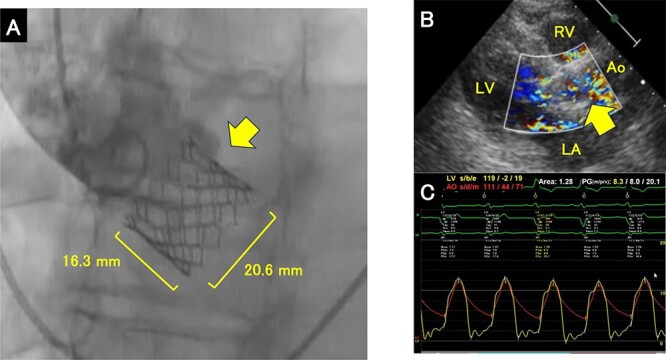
Images obtained after deployment of the THV. (A) Aortography shows 50/50 implantation as well as the height and diameter of the THV. The height is measured from the top to bottom of the stent. The diameter is measured from the right to left side of the stent. The arrow indicates the THV. (B) Transthoracic echocardiogram shows that the THV stayed in the aortic annulus. The arrow indicates the THV. (C) Pressure measurement at the aorta and left ventricle. THV; transcatheter heart valve, LA; left atrium, LV; left ventricle, Ao; Aorta.
